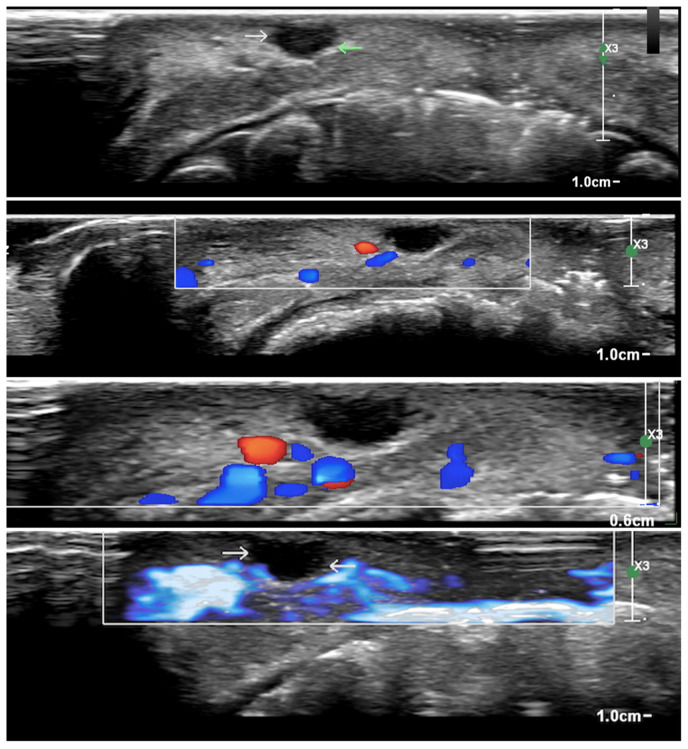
Extracts from the echocolor Doppler images. The ultrasound examination demonstrates the presence of a transonic, oval-shaped mass in the lower lip, with well-defined and sharp contours. No evidence of intralesional vascularization is observed. Blu areas refer to small venous vessels, red areas to small arterial vessels. In the four ultrasound images, a transonic mass with sharp and well-defined contours is clearly visible, lacking vascularization on color power Doppler interrogation. Peripheral vascularization is present, with a more significant vascular pole on the lateral right side. The role of ultrasound imaging in the study of soft tissues is well known. The examination uses ultrasound waves, physical elements that have no pathological effects on the patient or the operator. This method allows for the exploration of various regions of the human body. At the level of the lips and tongue, it is possible to examine any masses both in terms of tissue structure and, with the aid of color power Doppler, the vascularization of the lesions [27].
